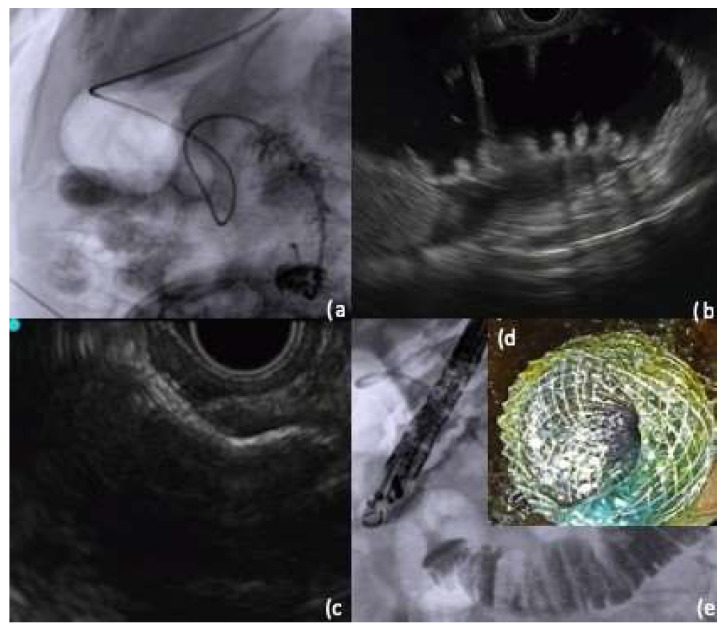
Gastroenteroanastomosis using lumen apposing metal stent (LAMS) with nasobiliary drain-assisted technique; (a) nasobiliary tube placement through the stenosis under fluoroscopic control; (b) endoscopic ultrasound (EUS) image of the target jejunal loop distended using fluid administration through the nasobiliary tube; (c) EUS image of first flange release of the LAMS; (d) final endoscopic image with methylene blue coming from the jejunum through the LAMS; (e) fluoroscopic image with contrast medium flowing freely from the gastric cavity to the jejunal loop through the LAMS. From the personal archive of the corresponding author.
